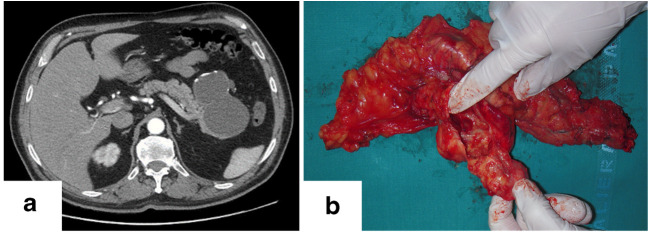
Computed tomography scan (a) and gross examination (b) showing a large unilocular cystic mass within the pancreatic body (diameter 8.7 cm)
